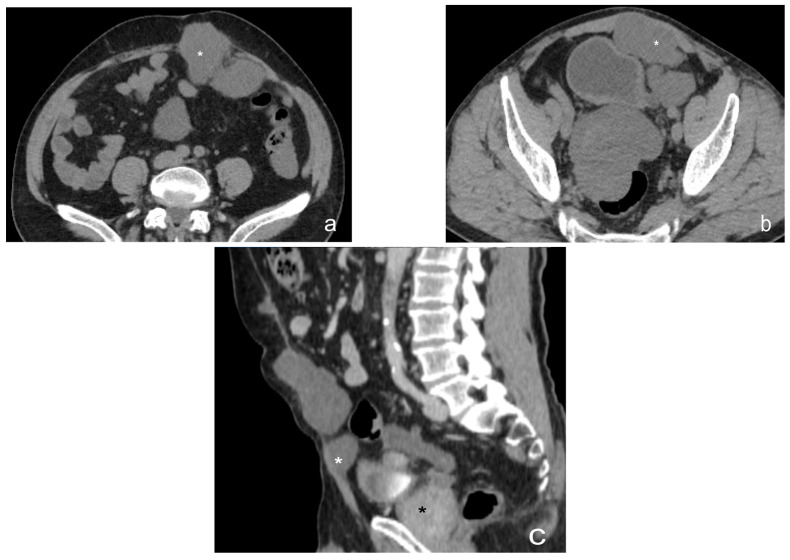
Contrast-enhanced CT scan, axial view (a,b) and sagittal view (c). Case of pseudomyxoma peritonei in a male, 68 y with left-colon adenocarcinoma, IV stage. Multiple peritoneal nodules (white star) involving the anterior abdominal wall (a–c) and a major nodule (black star) localized in pelvis, compressing the sigma.
